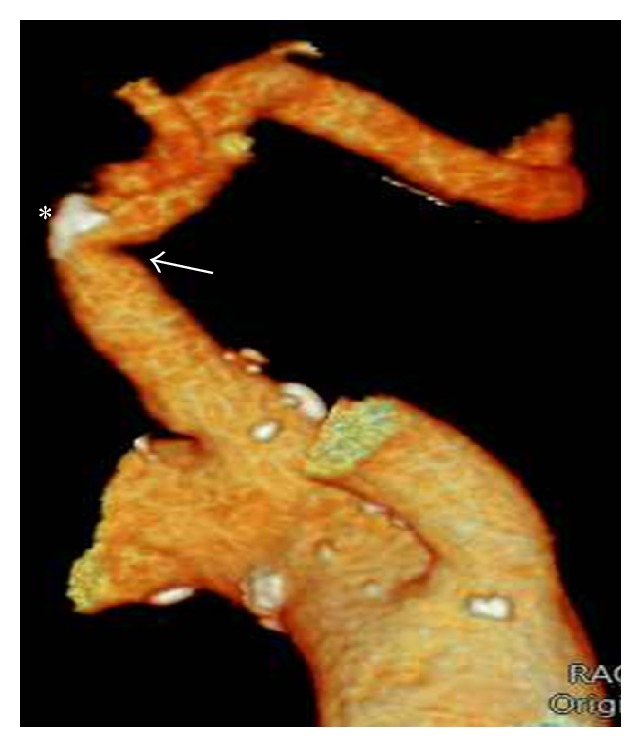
Computed tomography angiography revealing calcified plaque (asterisk) and a kink (arrow) just before the atherosclerotic plaque.
